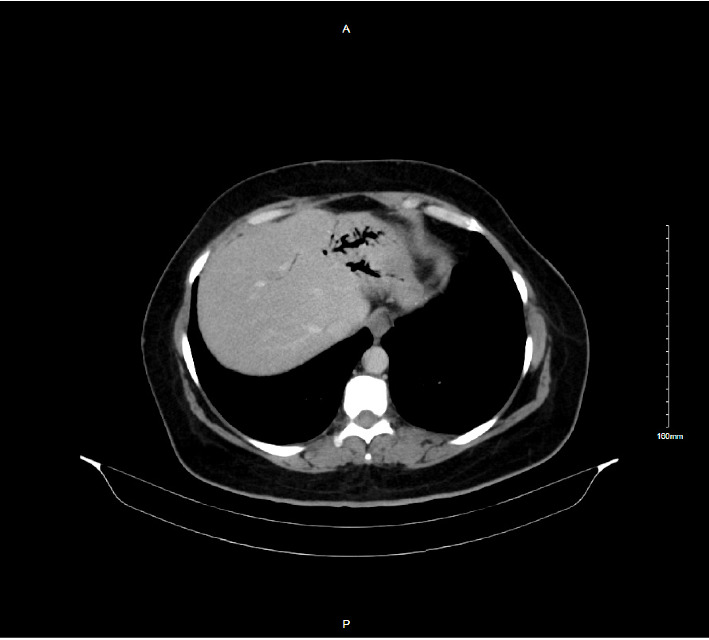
Abdominal CT scan (transverse section) demonstrating air within the left hepatic duct (pneumobilia).
